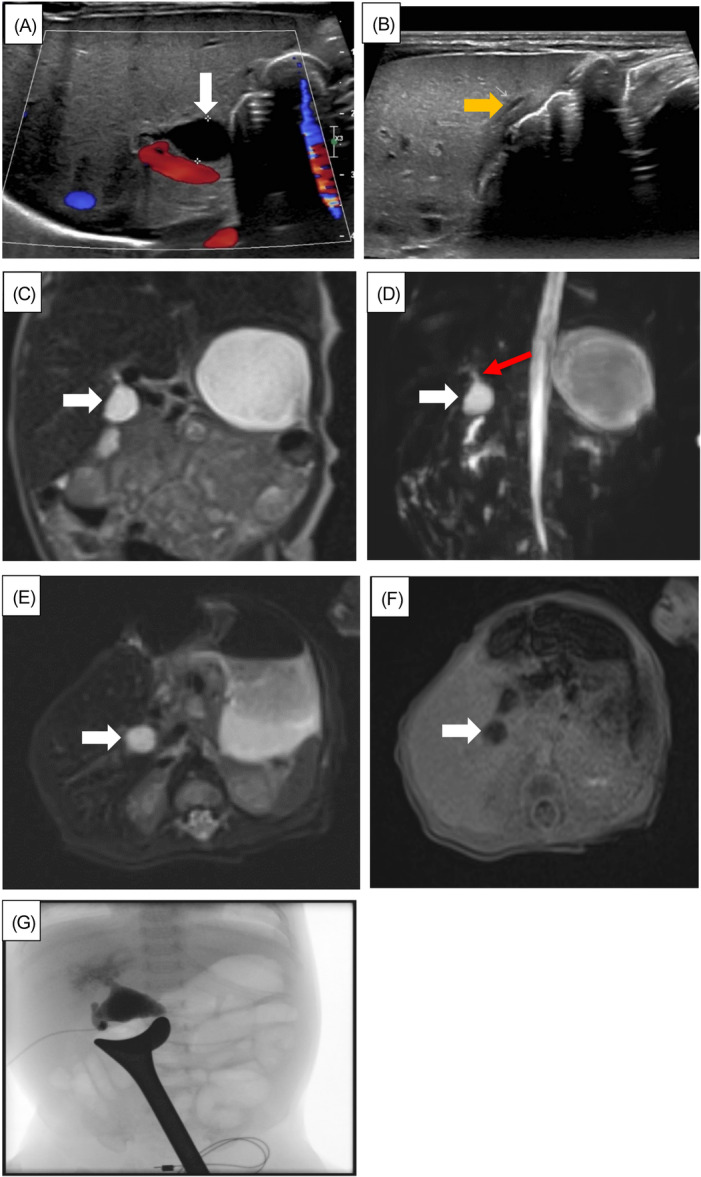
(A) Ultrasound Doppler of the liver revealing cystic structure in the porta hepatitis in continuity with the nondilated intrahepatic biliary system (white arrow). (B) Ultrasound Doppler of the liver, revealing a small gallbladder (orange arrow) was also present, which can be seen in BA. (C,D) T2‐weighted image coronal image from MRCP (C) and 3D maximum intensity projection MRI (D) demonstrating the cystic structure in the portal hepatis, which appears to communicate with the common hepatic duct. (D) Red arrow annotates a small proximal bile duct measuring 1.3 mm. (E,F) Axial T2 fat‐saturated image (E) and axial T1 20 min postcontrast delay image (F) after administration of hepatobiliary contrast agent, Eovist. The cystic structure remains dark on T1 and fails to opacify with excreted contrast. (G) Intraoperative cholangiography revealing that the gallbladder communicates with the proximal cystic structure that communicates with the left and right hepatic ducts. No evidence of distal flow into the duodenum is noted, suggestive of cystic variant of BA. 3D, three‐dimensional; BA, biliary atresia; MRCP, magnetic resonance cholangiopancreatography; MRI, magnetic resonance imaging.
